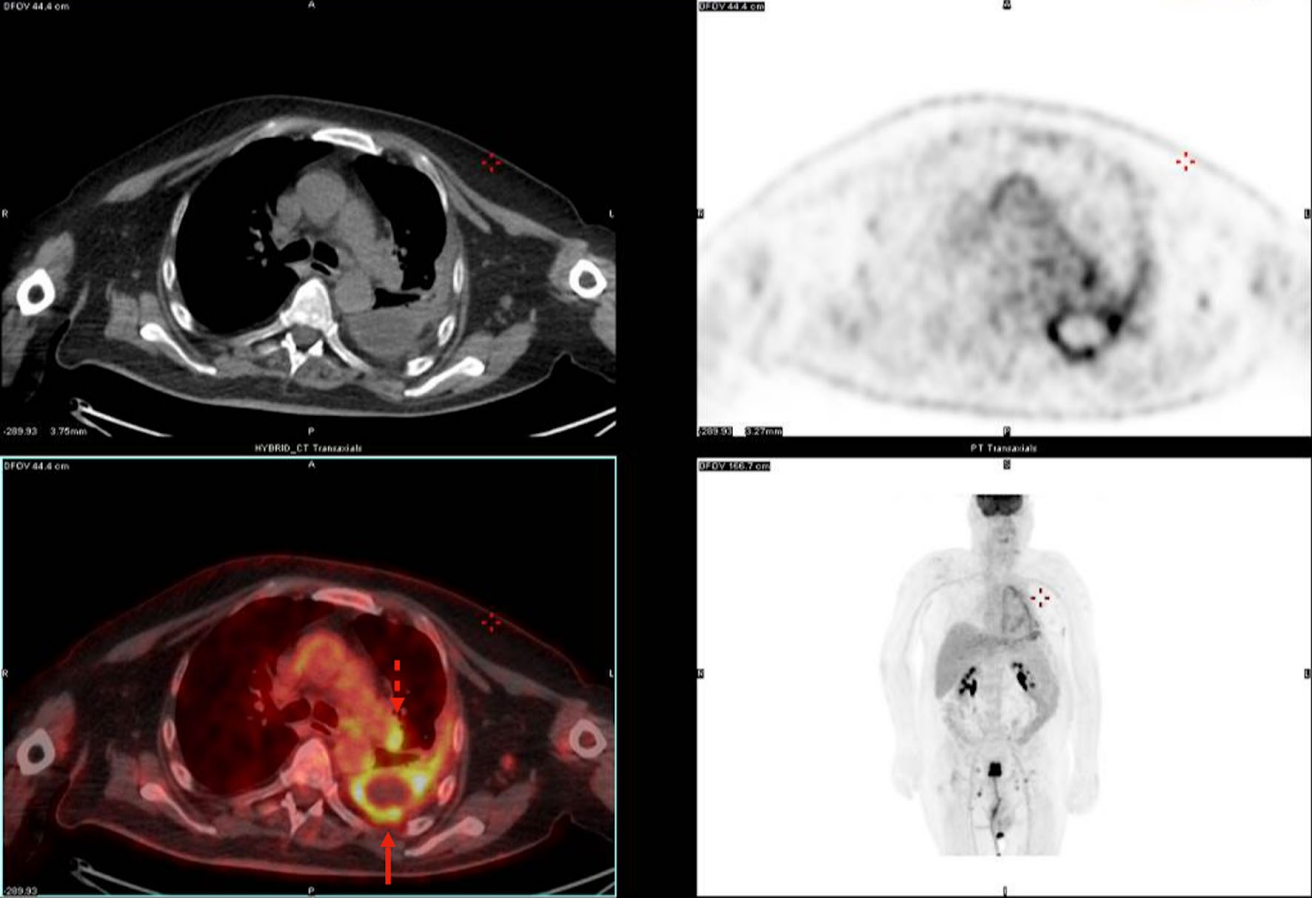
63-year-old man undergoing ^18^F-FDG PET/CT examination for PM staging. Pleural thickening (arrow) surrounding an area of loculated pleural effusion can be seen, along with ipsilateral hilar lymphadenopathy (dashed arrow)
